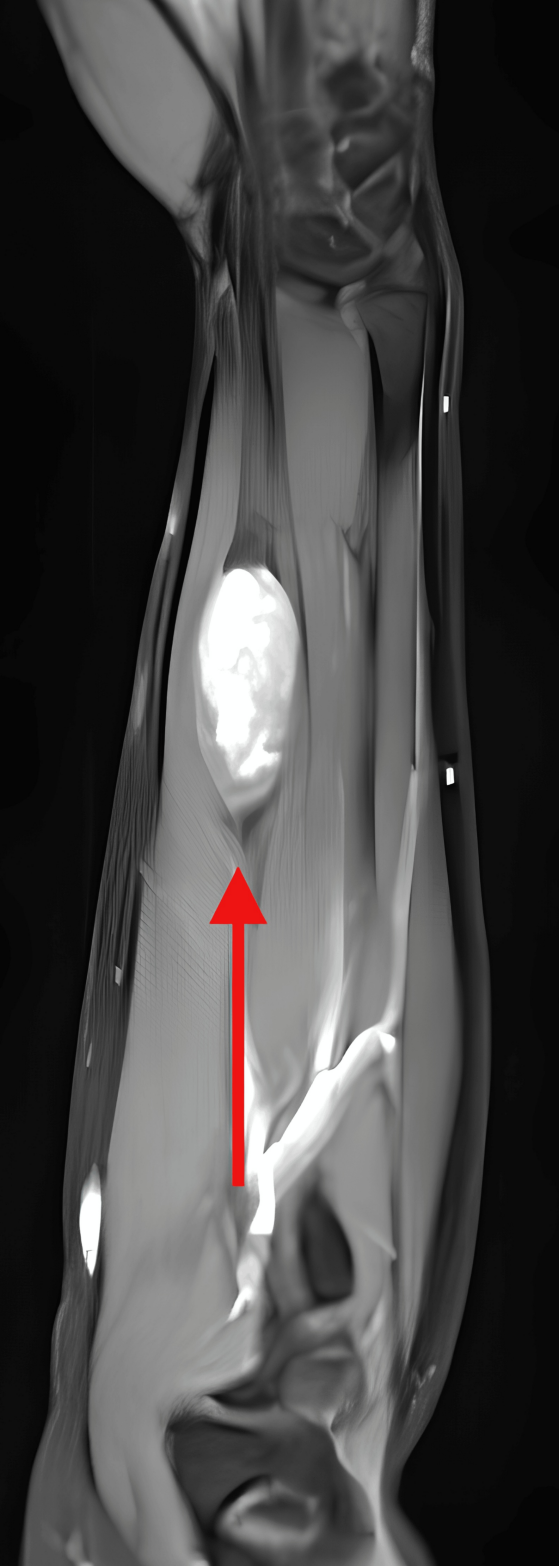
Fat-suppressed sagittal MRI image of the right forearm The red arrow shows a well-defined, oval-shaped, non-fat-suppressed, heterogeneous hyperintense lesion in the volar aspect of the distal right forearm, with no infiltration into the surrounding tissues.
